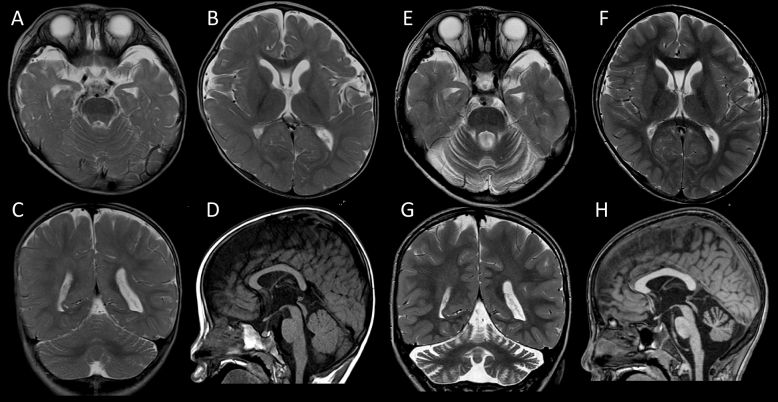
MRI of the brain at 2 years (A-D) and 7 years old (E-H) with axial T2WI (A, B, E, F). Coronal T2WI (C, G) and sagittal T1WI (D, H). MRI at 2 years old, demonstrating persistent delayed myelination. However, by 7 years of age, cerebellar hemisphere and vermis atrophy became apparent, despite the absence of clinical signs of ataxia. Cerebral myelination appeared normal at that time. MRI: magnetic resonance imaging.
